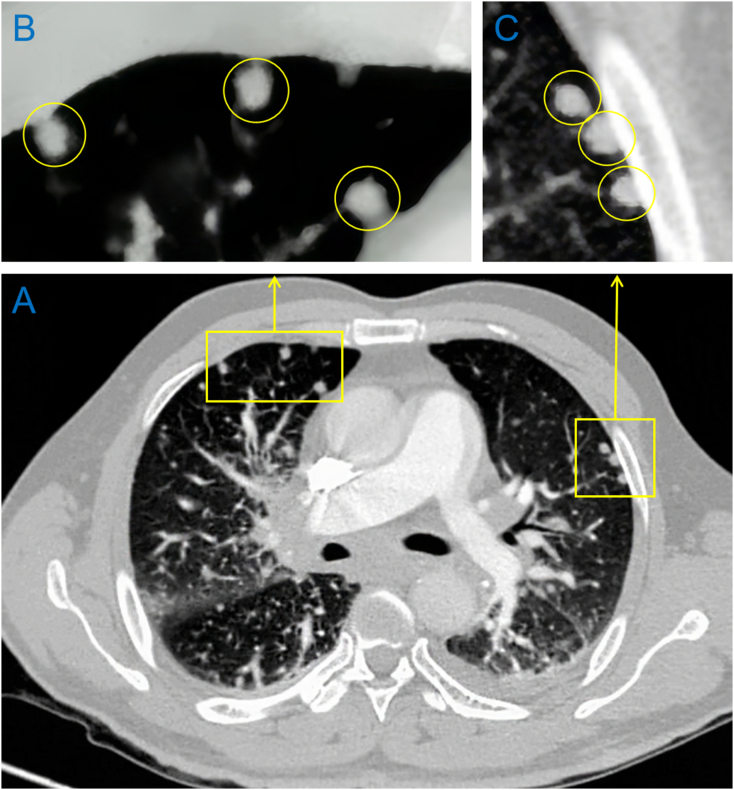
The CTA chest of patient 3 after thrombolytic therapy. A: multiple nodules in the lungs; B, C: zoom-in view of lung nodules.
